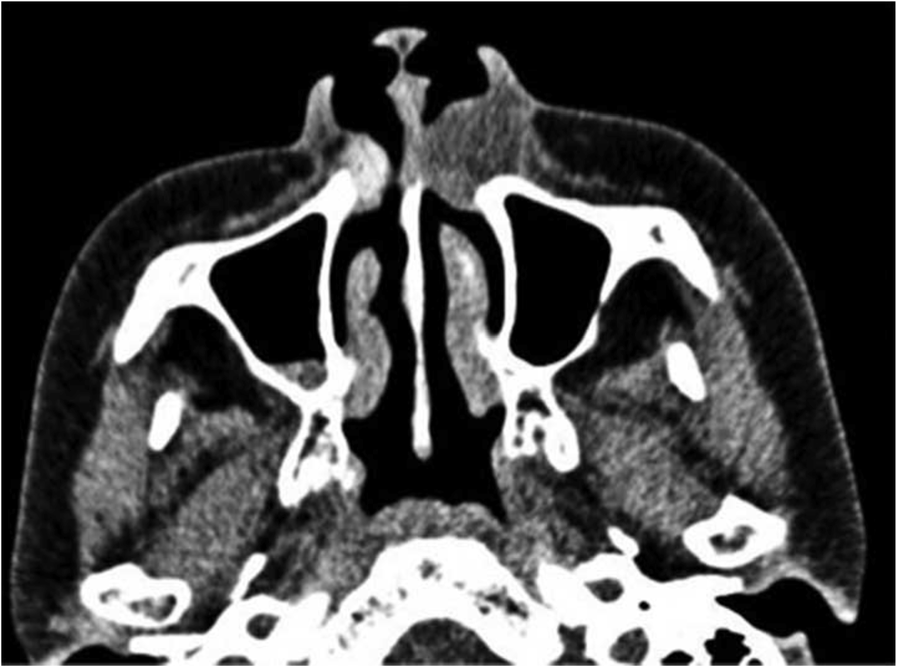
Computed tomography image obtained at the first examination. A well-circumscribed oval lesion, approximately 19 × 14 × 12 mm in size, was found inferior to the left nasal alar base. A small high-density area was observed adjacent to the right piriform aperture
